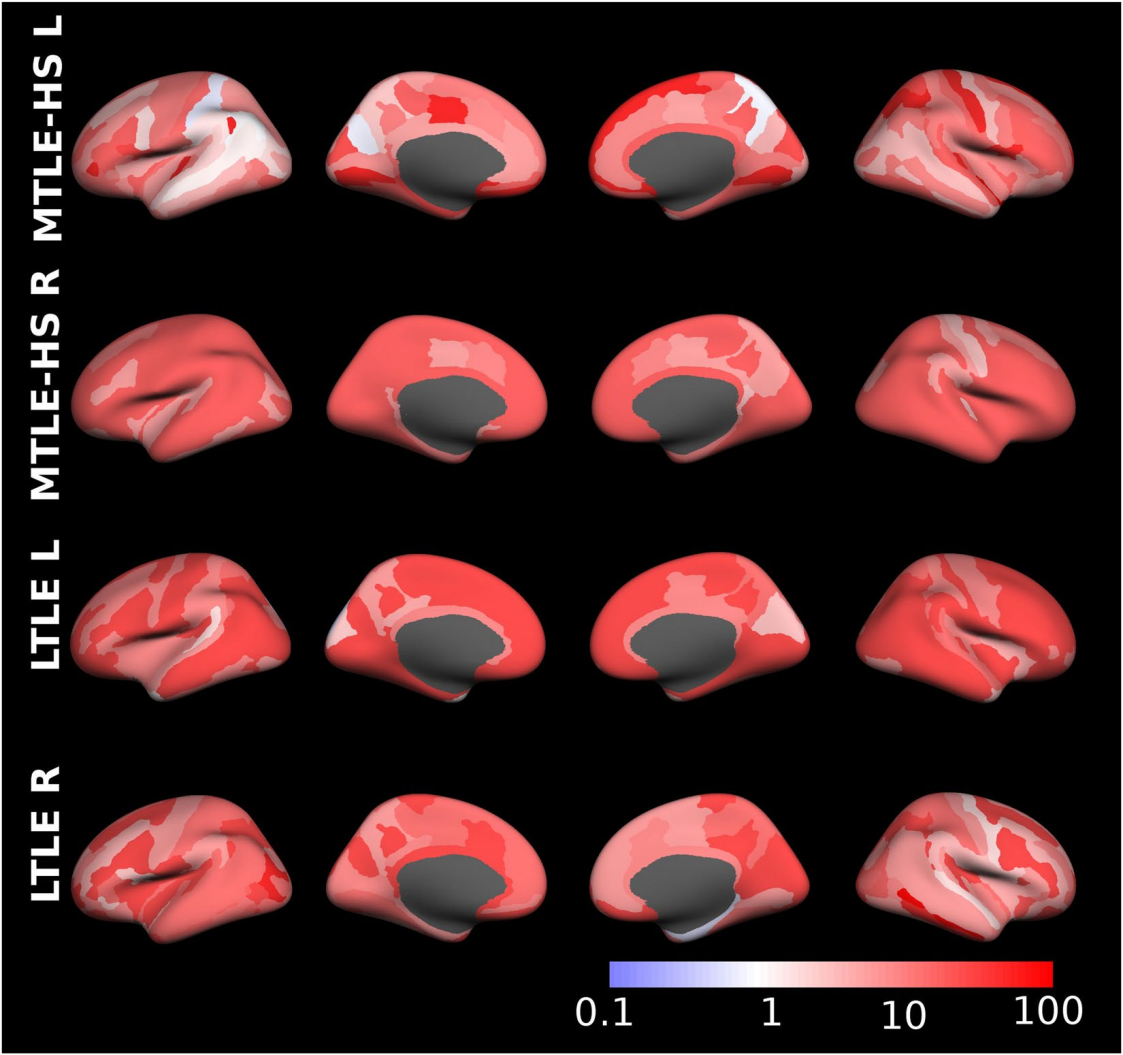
Diagnostic odds ratio of morphometric detections in surface parcellations with respect to expert MRI assessment. For better visibility of sulci the results are presented on inflated brain surfaces.
